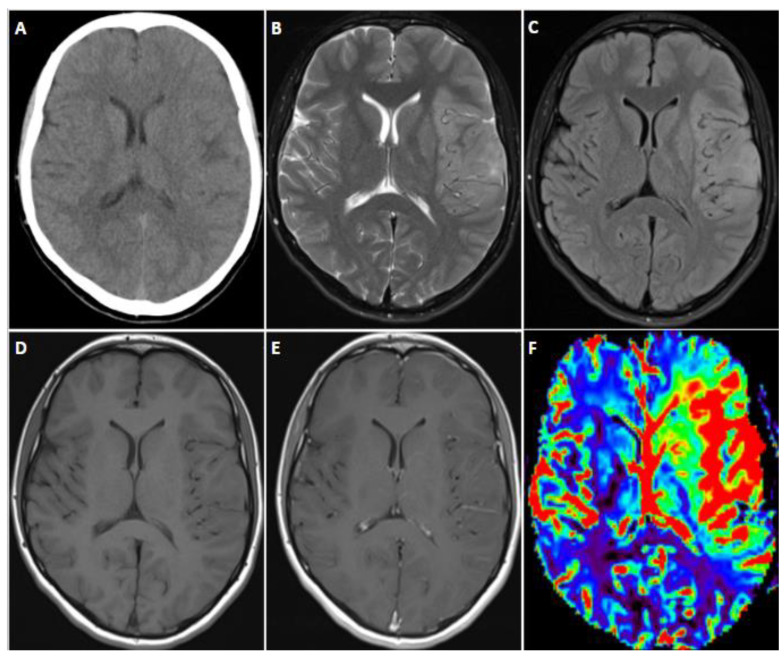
Axial unenhanced CT (A), axial T2- weighted (B), axial T2 fluid-attenuated inversion recovery (FLAIR) (C), axial T1-weighted (D), axial T1-weighted post-contrast (E), and cerebral blood volume map from dynamic susceptibility contrast (DSC) perfusion images (F) through the level of the lateral ventricles in a 22 year old female with diffuse hemispheric glioma, H3 G34-mutant and IDH-wildtype tumor, who presented with first-time seizure. The images demonstrate an infiltrative left frontal and left temporal operculum mass, as depicted by abnormal T2 FLAIR hyperintensity, with associated increased perfusion and no abnormal contrast enhancement. Lesion is unifocal with leptomeningeal contact at its lateral aspect. There was no diffusion abnormality. Note that this was initially misdiagnosed as acute encephalitis.
